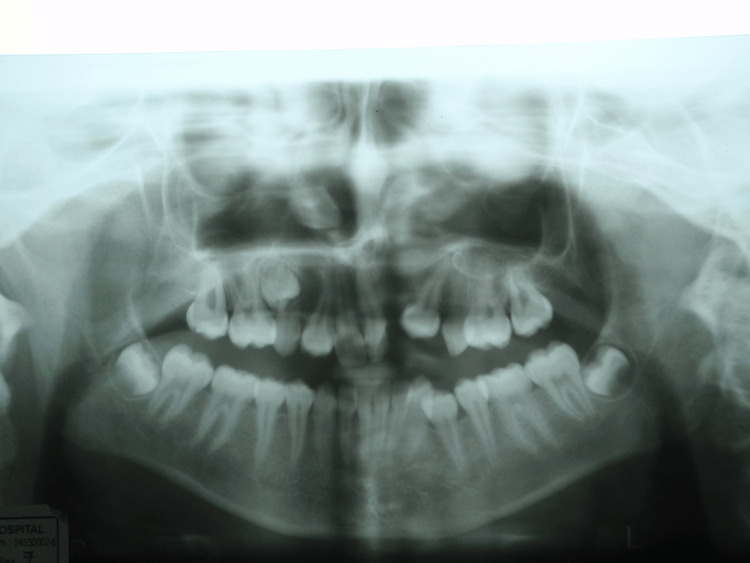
Orthopantomagram (OPG) reveals cleft in the alveolus with impacted 23.
